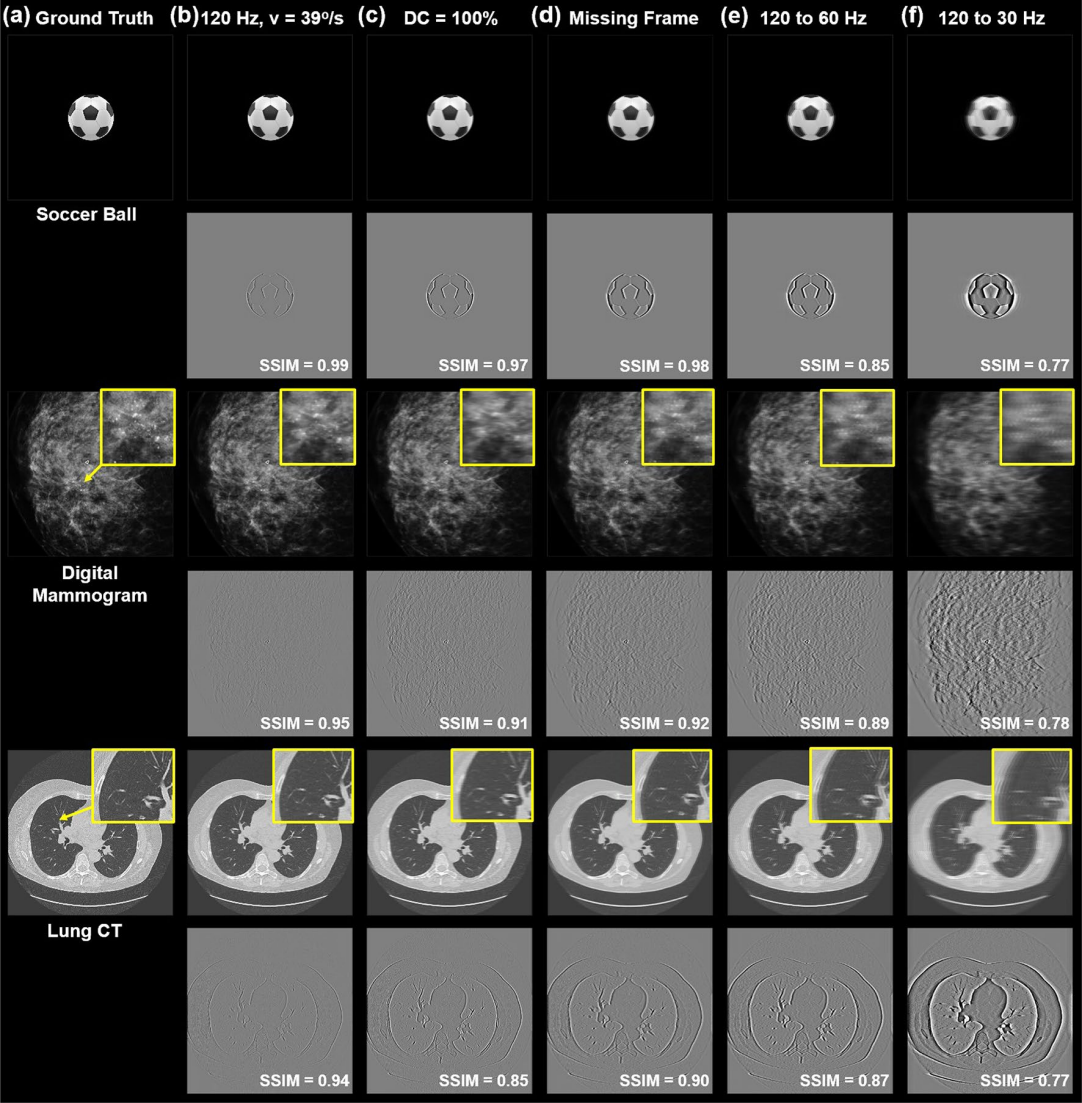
Visualizations of motion image artifacts and motion image errors (difference images with respect to static images) of a soccer ball (first row), a digital mammography image (second row) and a lung CT slice (third row) in motion: (a) referencing static images, (b) moving scenes at 39°/s using a pulsed 120 Hz refresh rate, (c) a continuous emission with 100% duty cycle, moving images with a missing frame (d) and frame rate reduction from 120 Hz to 60 Hz (e) and 30 Hz (f).
